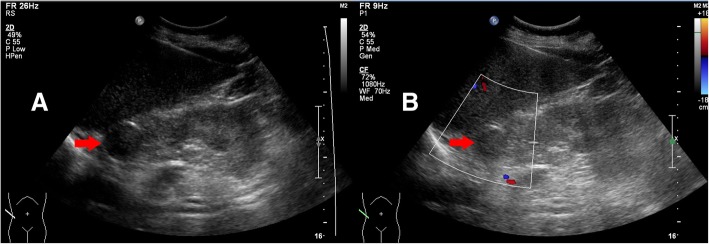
Examination of the adrenal gland by ultrasonography. a Two-dimensional ultrasonography: a mass 2.9 cm × 2.8 cm in size with a weak echo was found in the of right side of the adrenal gland. The boundary of the mass was clear. b Color doppler ultrasound: no blood flow signal was found in the adrenal gland mass
